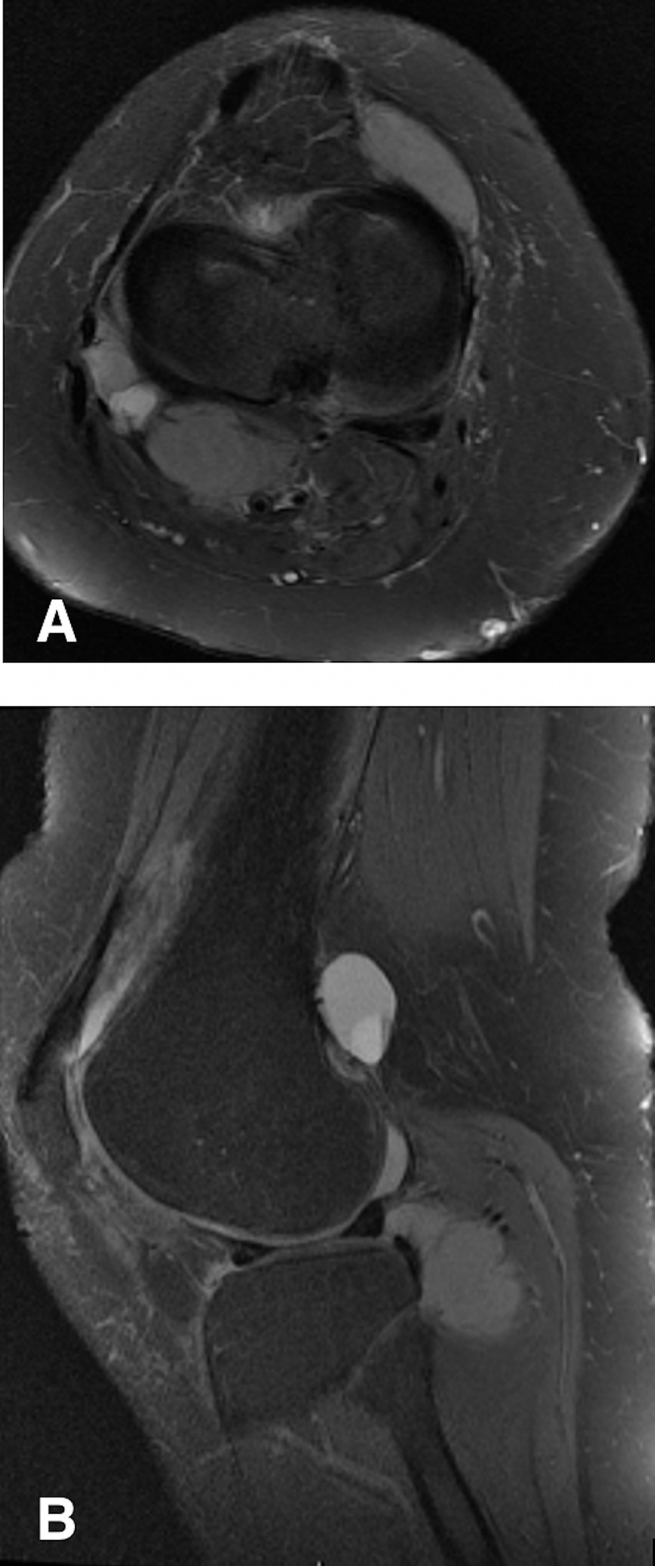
(a) T2-weighted, fat-suppressed axial, (b) proton density, fat-suppressed sagittal magnetic resonance images of the right knee in a 47-year-old woman (patient two). Images show multiple residual tumor lesions after previous arthroscopic debridement.
